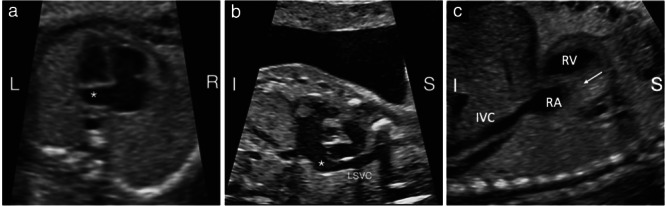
Ultrasound images from different fetuses in midgestation with single left superior vena cava (LSVC). (a) Apical cross‐section of the four‐chamber view in a slightly caudal plane demonstrating the dilated coronary sinus (
